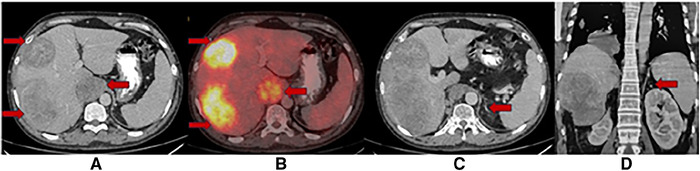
(A) Computed tomography (CT) axial image of the abdomen shows multiple liver metastases (arrows). (B) 18F-fluorodeoxyglucose (FDG) positron emission tomography/CT axial image of the abdomen shows multiple FDG-avid liver metastases (arrows). (C) CT axial image of the abdomen shows atrophied left adrenal gland (arrow). (D) CT coronal image of the abdomen shows atrophied left adrenal gland (arrow).
